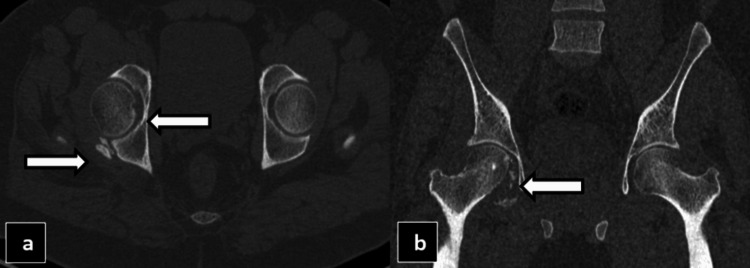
The CT pelvis axial reformatted image (a) and coronal reformatted image (b) in the bone window show comminuted displaced fractures involving the medial aspect of the articular surface of the right head of the femur with multiple adjacent bony fragments and comminuted fracture seen involving the posterior wall and posterior column of the right acetabulum (white arrows).
